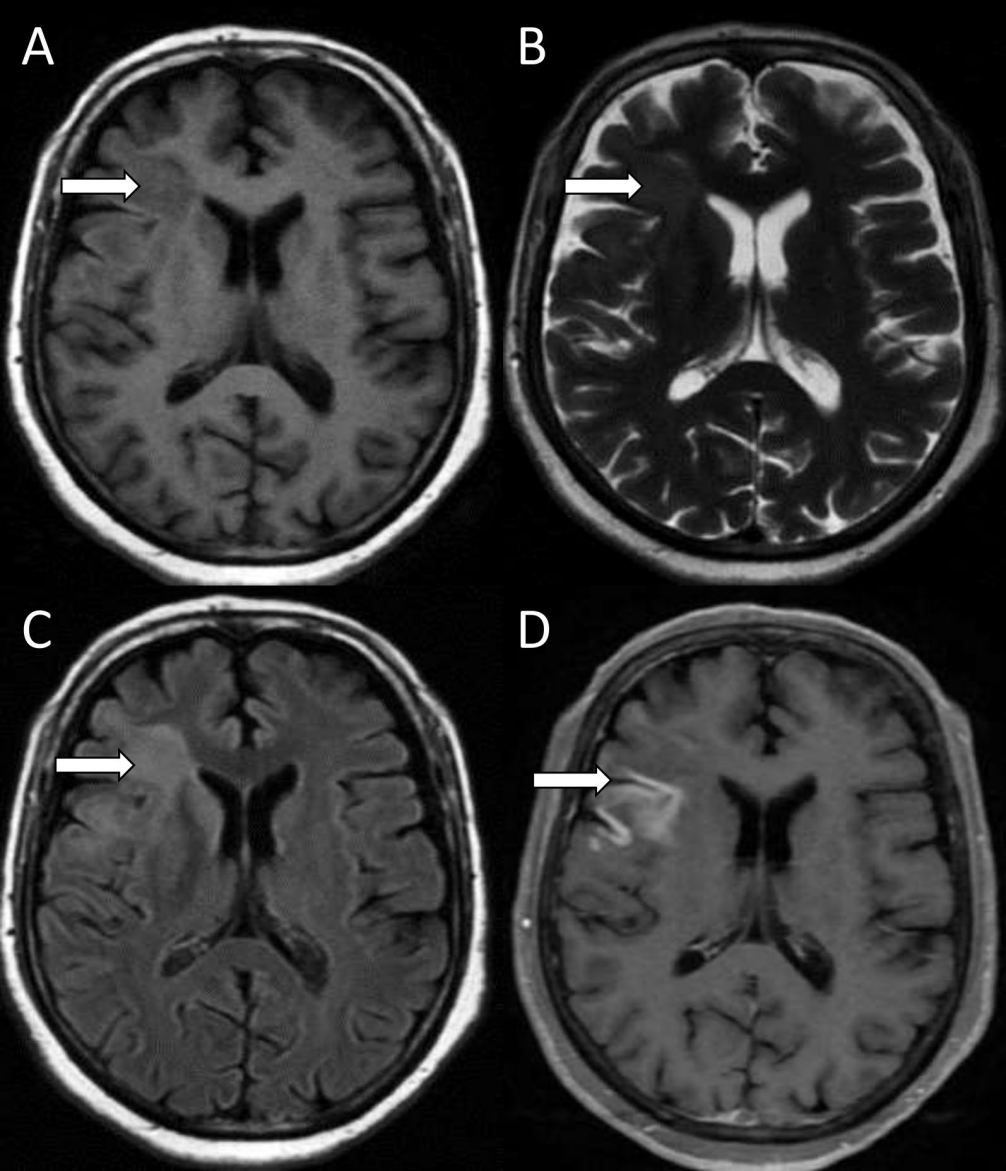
Rhinocerebral mucormycosis with cerebritis. Axial T1-weighted image (A) shows an isointense lesion in the right frontal lobe (white arrow). It appears hyperintense on axial T2-weighted (B) and FLAIR (C) images and shows enhancement on contrast-enhanced axial T1-weighted fat-saturated image (D)
